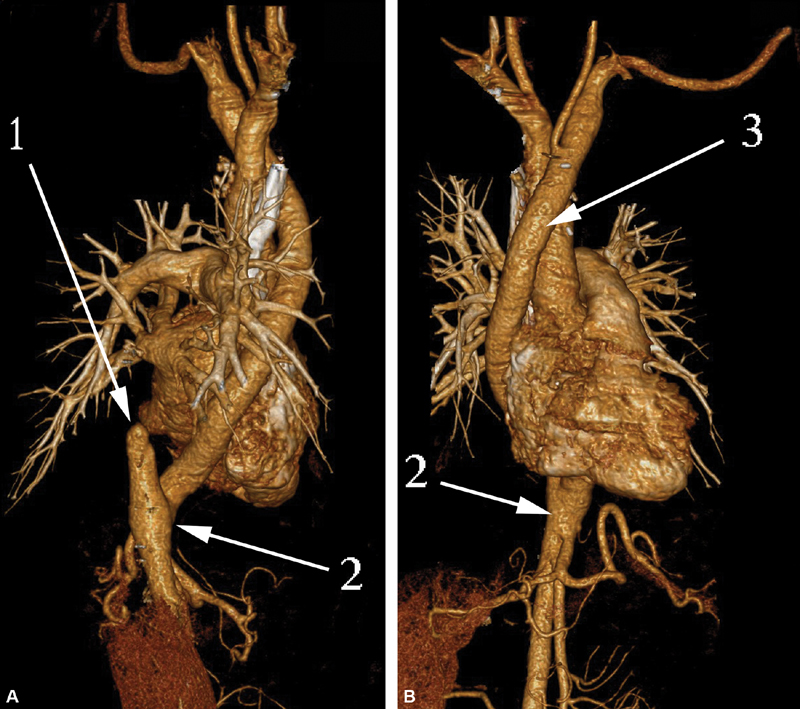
Computed tomography volume rendering lateral view (
